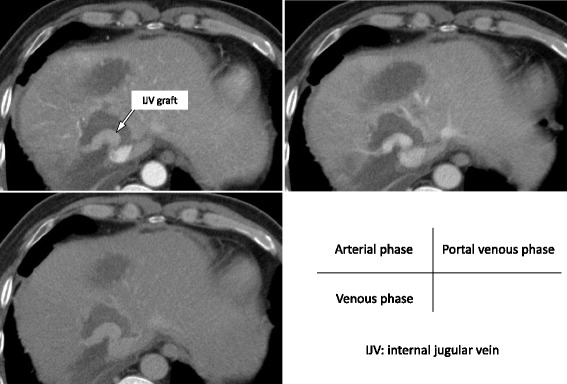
Enhanced computed tomography image of the liver in three phases on postoperative 7. The reconstructed hepatic vein was patent (*arrow*), and there was no hepatic congestion or ascites. *IJV* internal jugular vein
